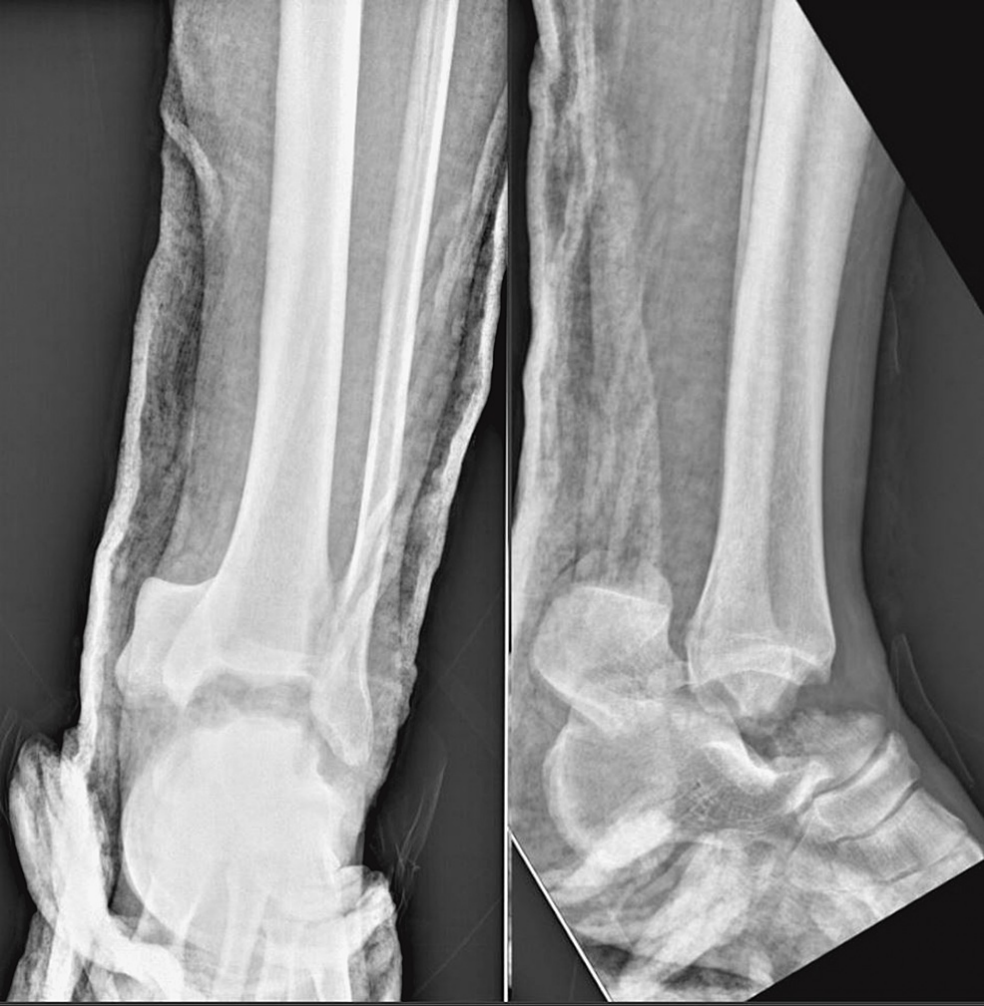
Anteroposterior (AP) and lateral X-rays of the left ankle
